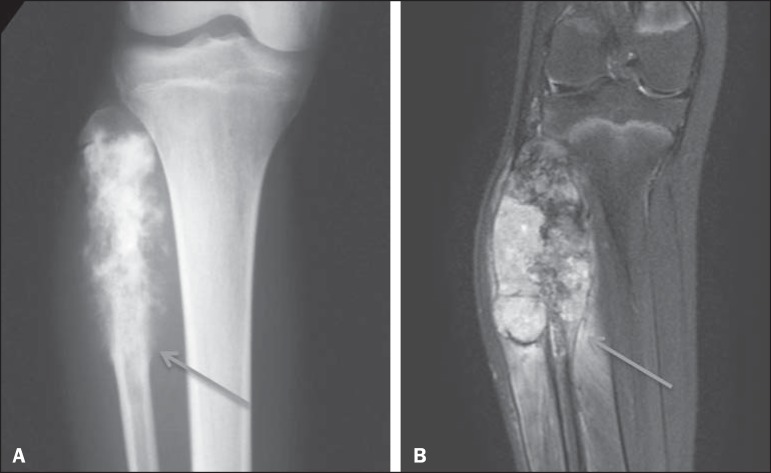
Codman's triangle, identified by the observers only on the MRI scan
